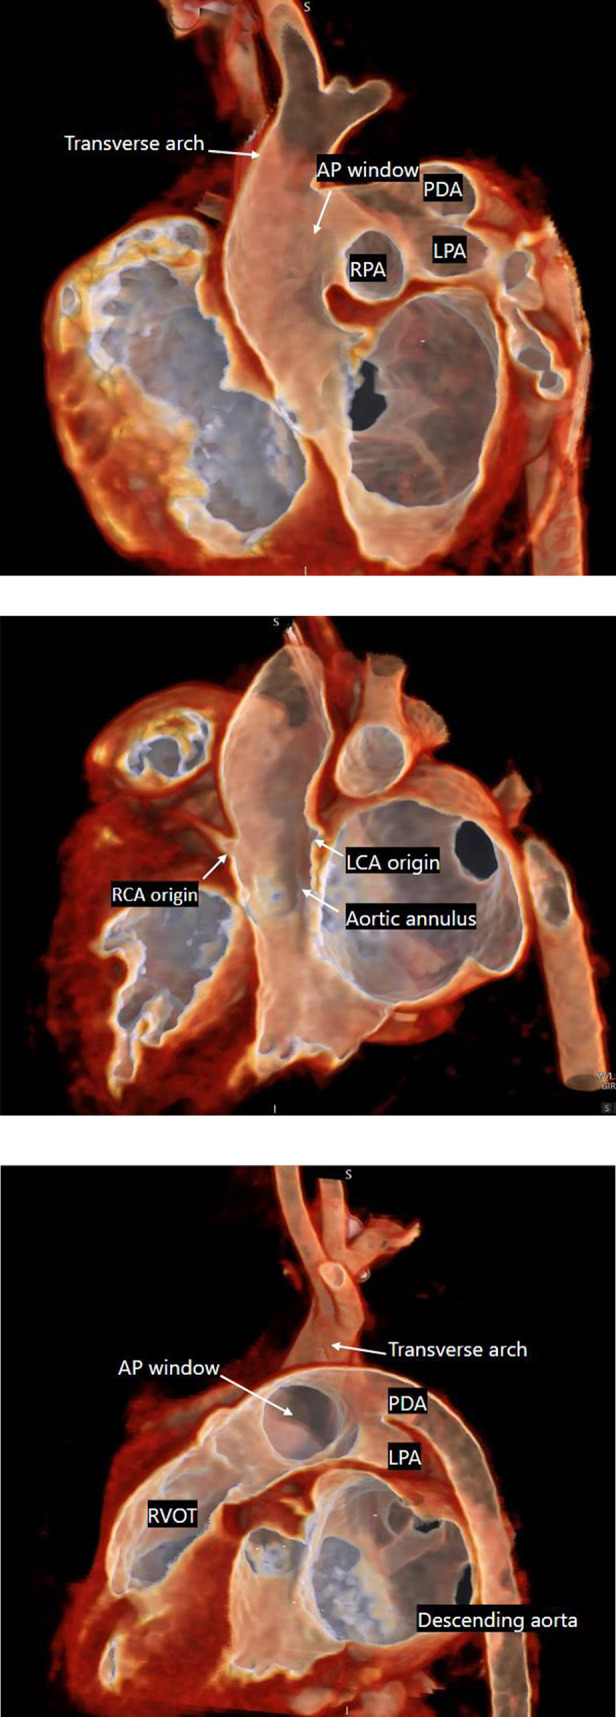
A. CTA image large APW in close proximity to transverse arch. B. CTA image identifying high origins of coronary arteries, above the level of the annulus. C. CTA image revealing distance between descending aorta and transverse aorta.
